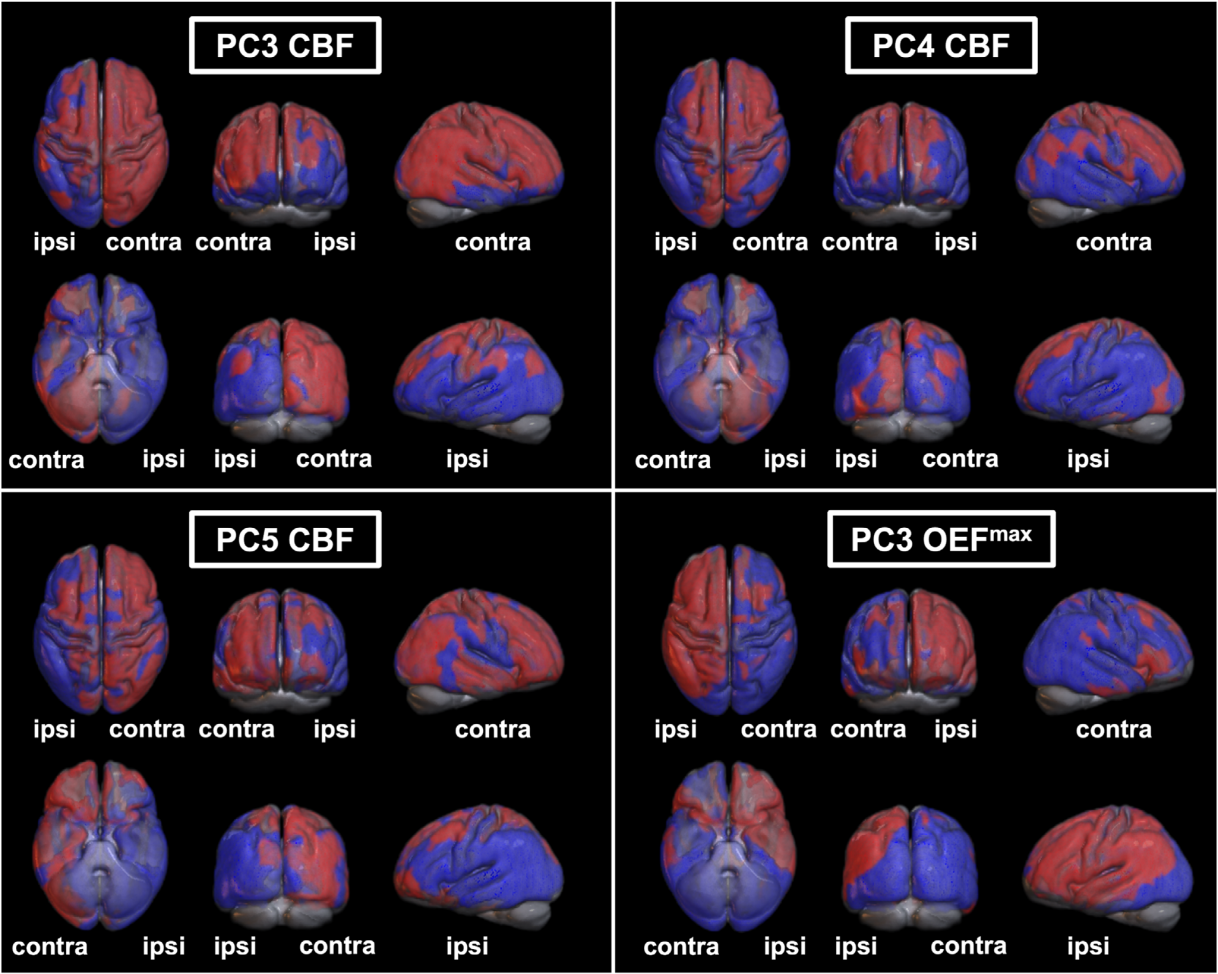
Topography of individual principal components (PCs) distinguishing ICAS from HC. Three‐dimensional renderings of binarized PCs overlaid on the mean T_1_‐weighted MRI of all participants. Ipsi‐/contralateral hemispheres are marked accordingly. Regions with positive loadings are displayed in red, while regions with negative loadings are shown in blue. For CBF, PC3 (top left), PC4 (top right), and PC5 (bottom left) yielded significantly different scores between ICAS and HC, whereas only PC3 separated ICAS from HC for OEF^max^.
